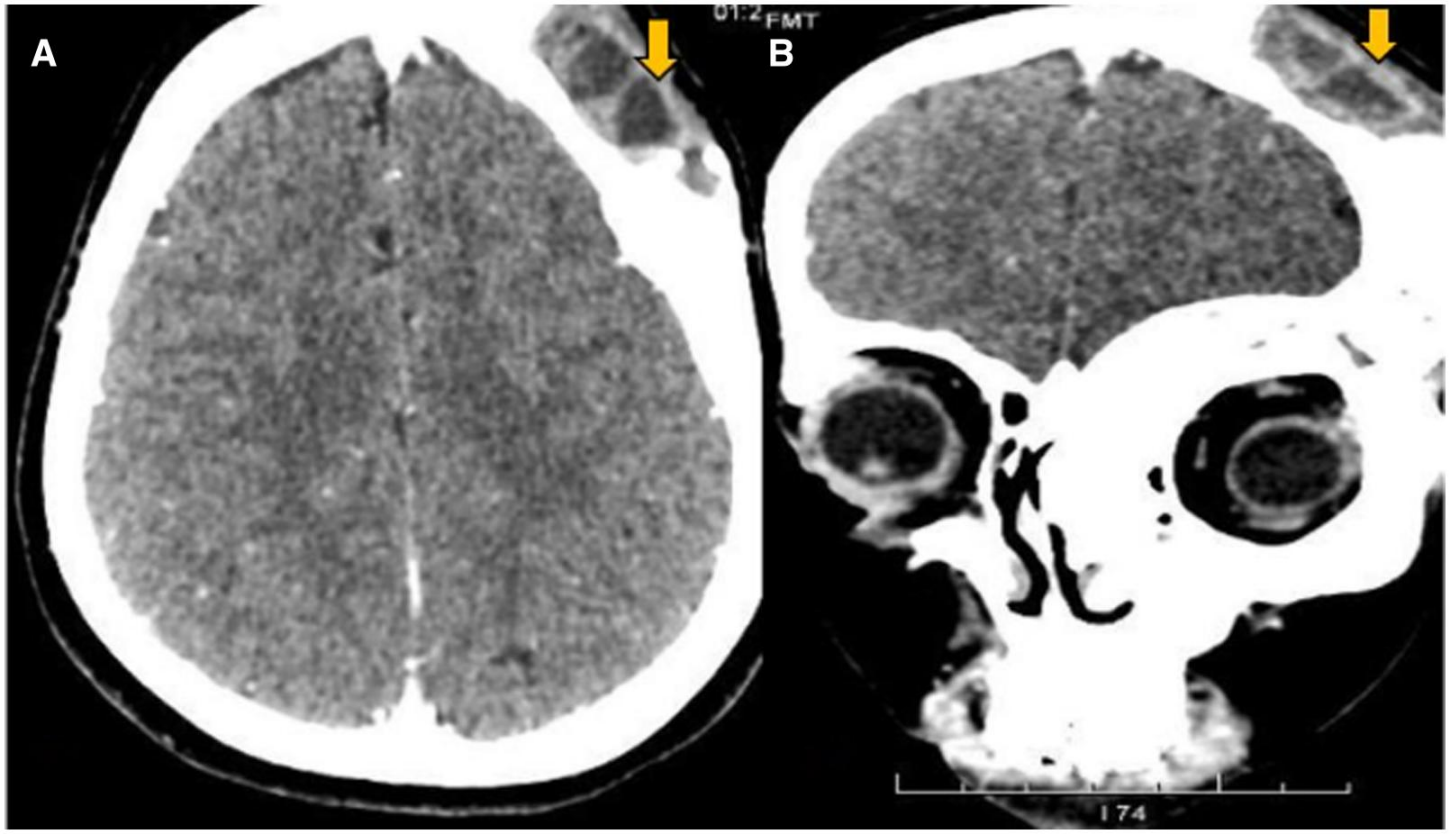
(A) CT brain axial plane soft tissue window showing focal soft tissue and cystic component seen in left frontal bone with osteolytic lesion and blood-fluid level. (B) CT brain coronal plane soft tissue window showing focal soft tissue and cystic component seen in left frontal bone with osteolytic lesion and blood-fluid level.
